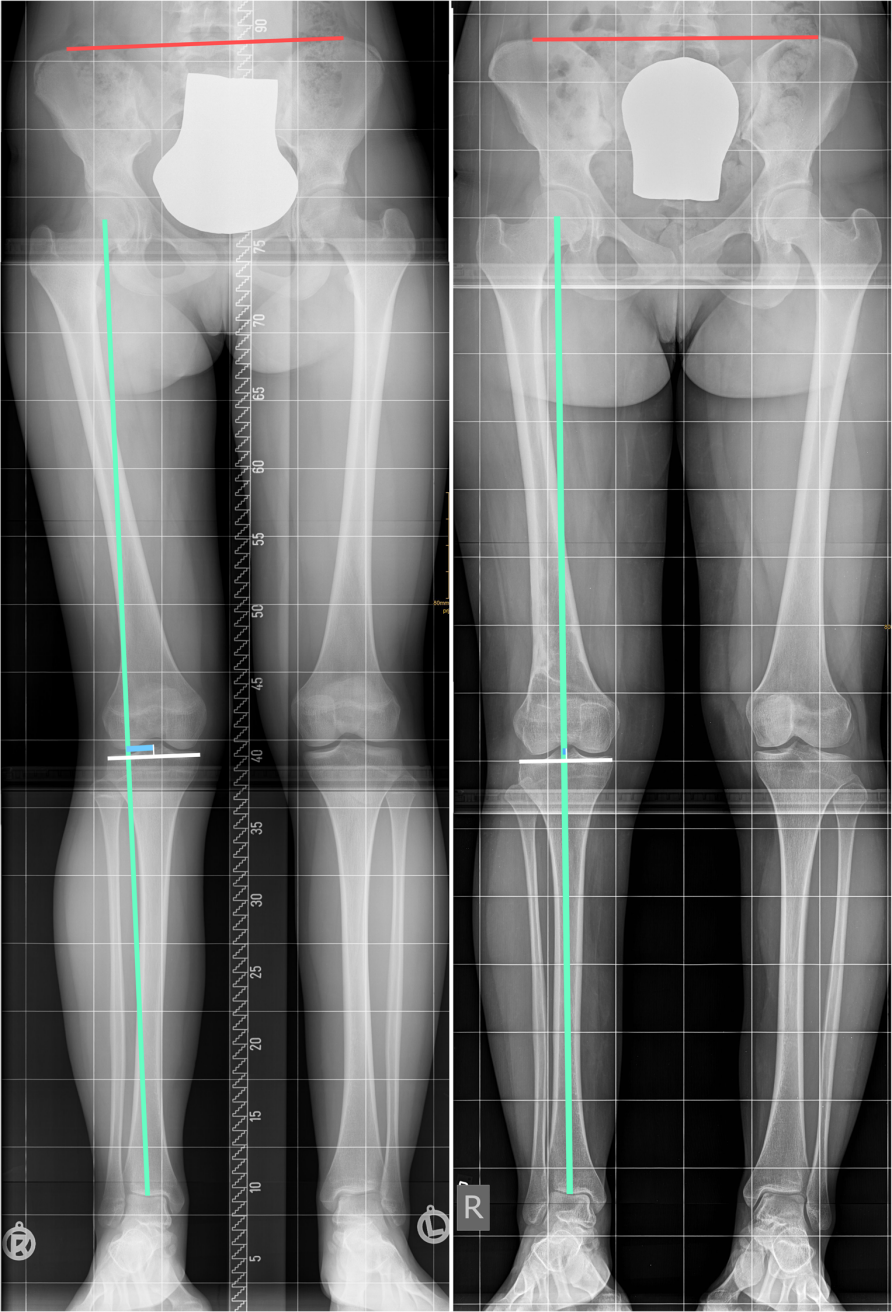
Comparison of pre- and post-operative long leg standing radiographs: mechanical axis of the leg (green line), tibial plateau width (white line with mark at the midpoint), MAD (blue line), and LLD (red line) radiographs
